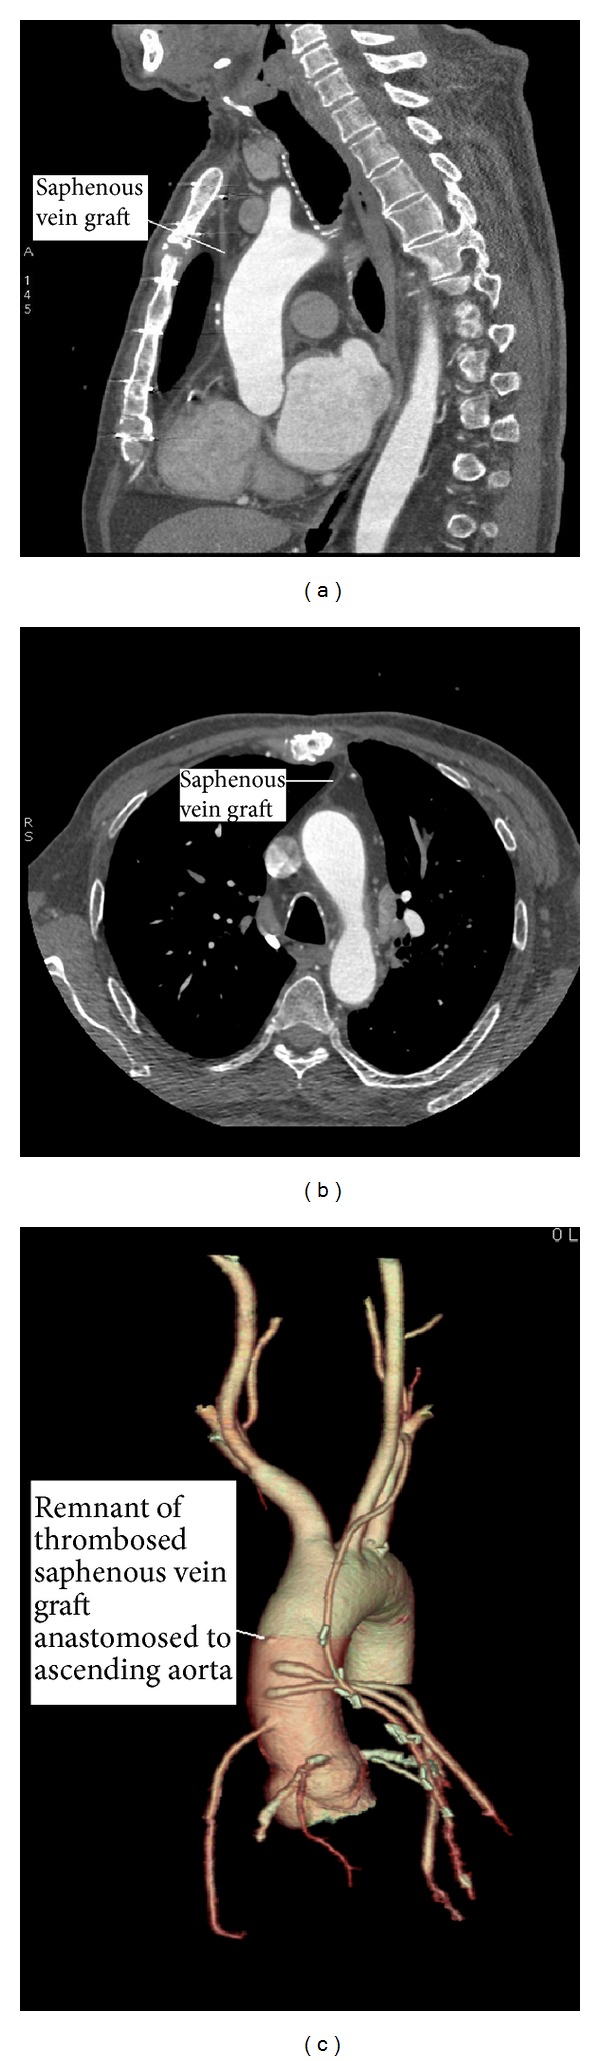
At postoperative 6th month, multislice CT imaging shows thrombotic occlusion at the remnant of the saphenous vein graft. (a) Sagittal section imaging of thrombosed saphenous vein graft. (b) Cross-sectional appearance of thrombosed saphenous vein graft. (c) Three-dimensional display of remnant of thrombosed saphenous vein graft anastomosed aorta.
